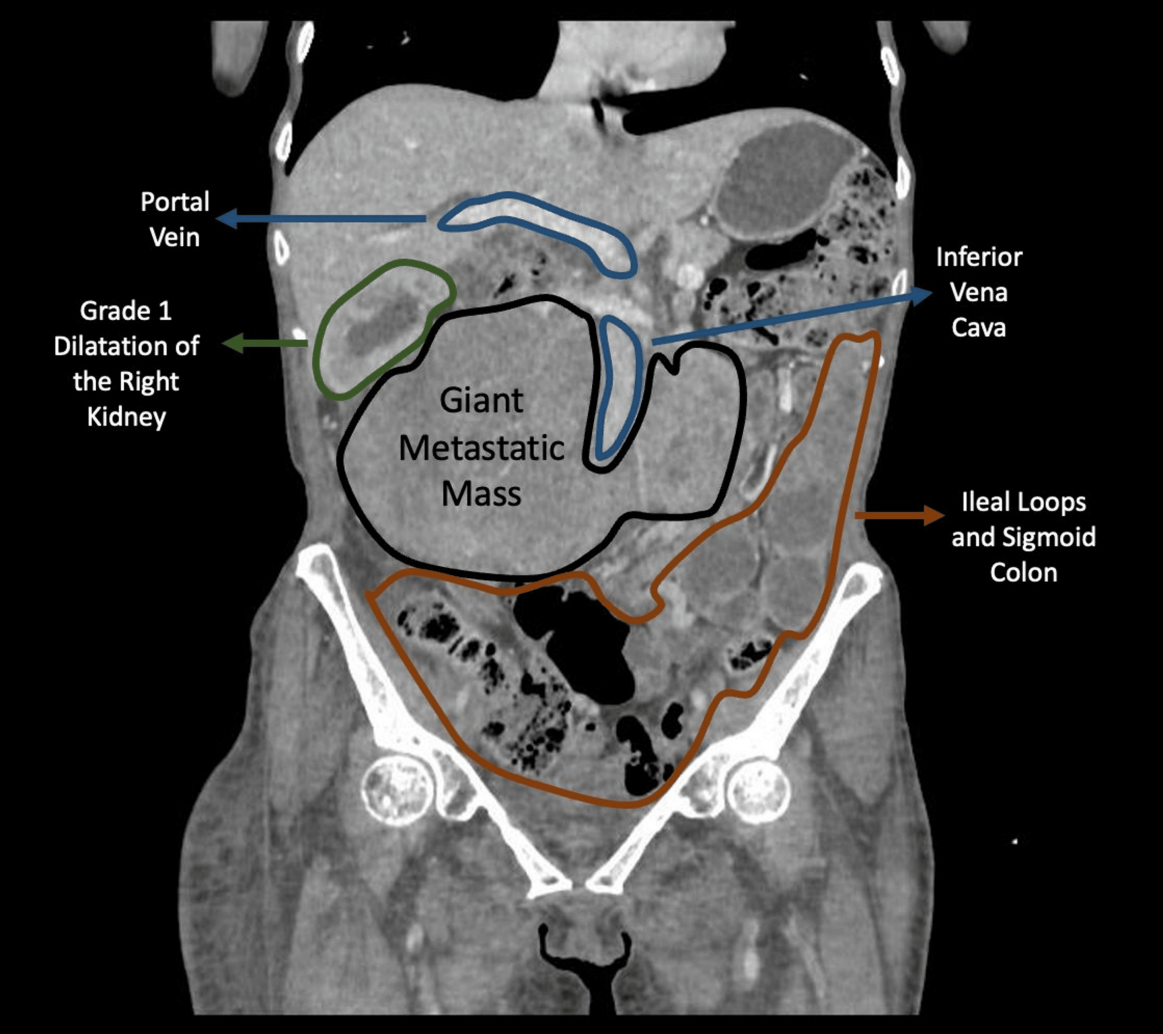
Contrast-enhanced coronal CT image demonstrating a large retroperitoneal mass Post-contrast coronal image demonstrating an enlarged mass encasing the aorta and dispşacing the right kidney superiorly
